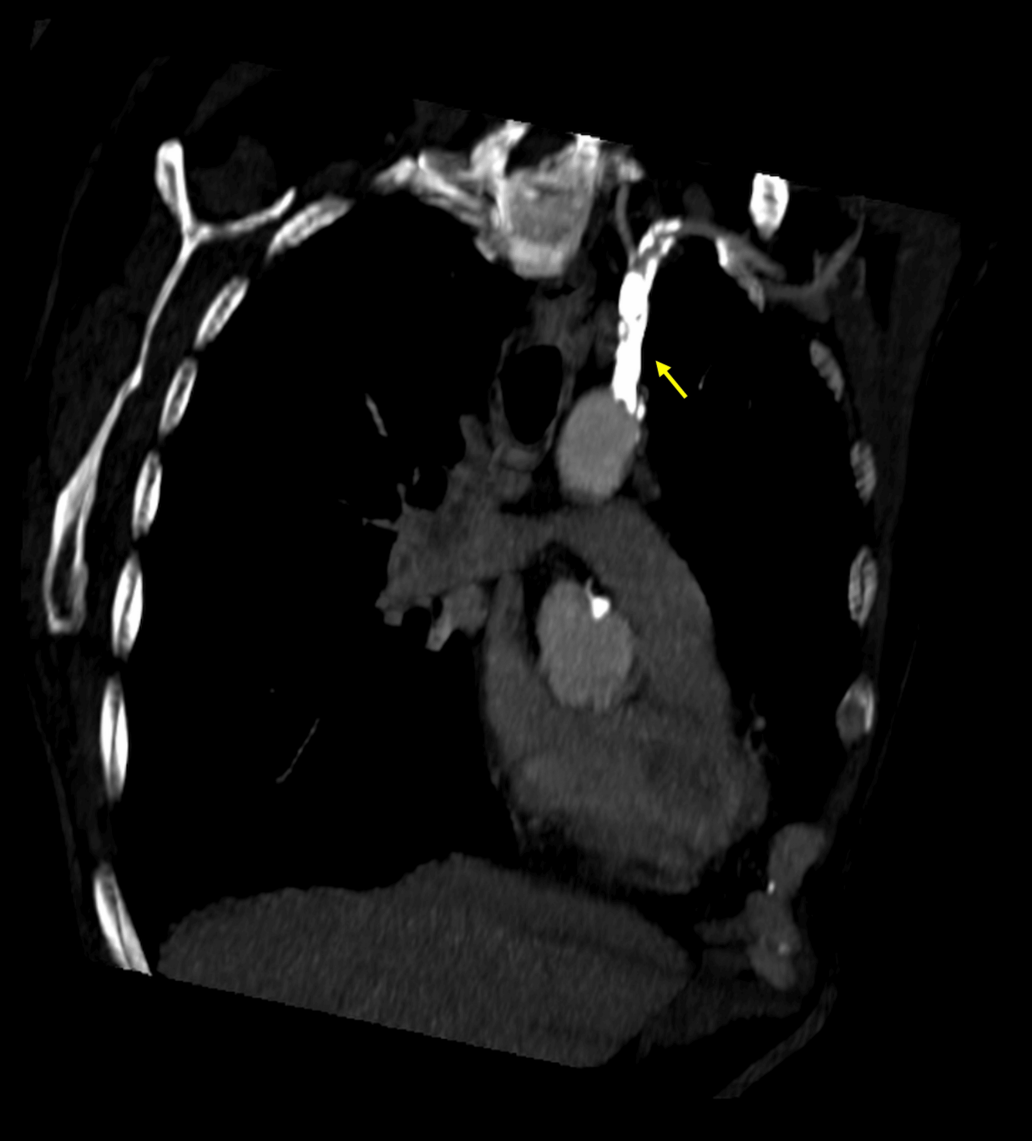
Coronal CT scan with the origin of brachiocephalic trunk and left subclavian artery with arteriosclerosis calcifications (yellow arrow)
